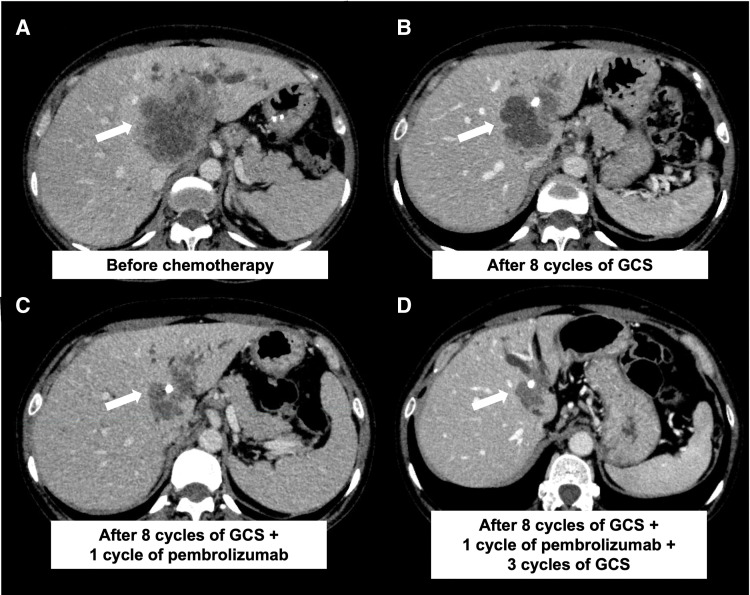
Abdominal CECT during chemotherapy. (A) Before starting chemotherapy, CECT showed a 6.5 × 5.2 cm tumor (arrow). (B) After 8 cycles of GCS chemotherapy, CECT showed a 5.0 × 3.4 cm tumor (arrow). (C) After 1 cycle of pembrolizumab therapy, CECT showed a 4.9 × 3.0 cm tumor (arrow). (D) After another 3 cycles of GCS therapy, CECT showed a 2.7 × 2.1 cm tumor (arrow). CECT, contrast-enhanced CT; GCS, gemcitabine, cisplatin plus S-1
